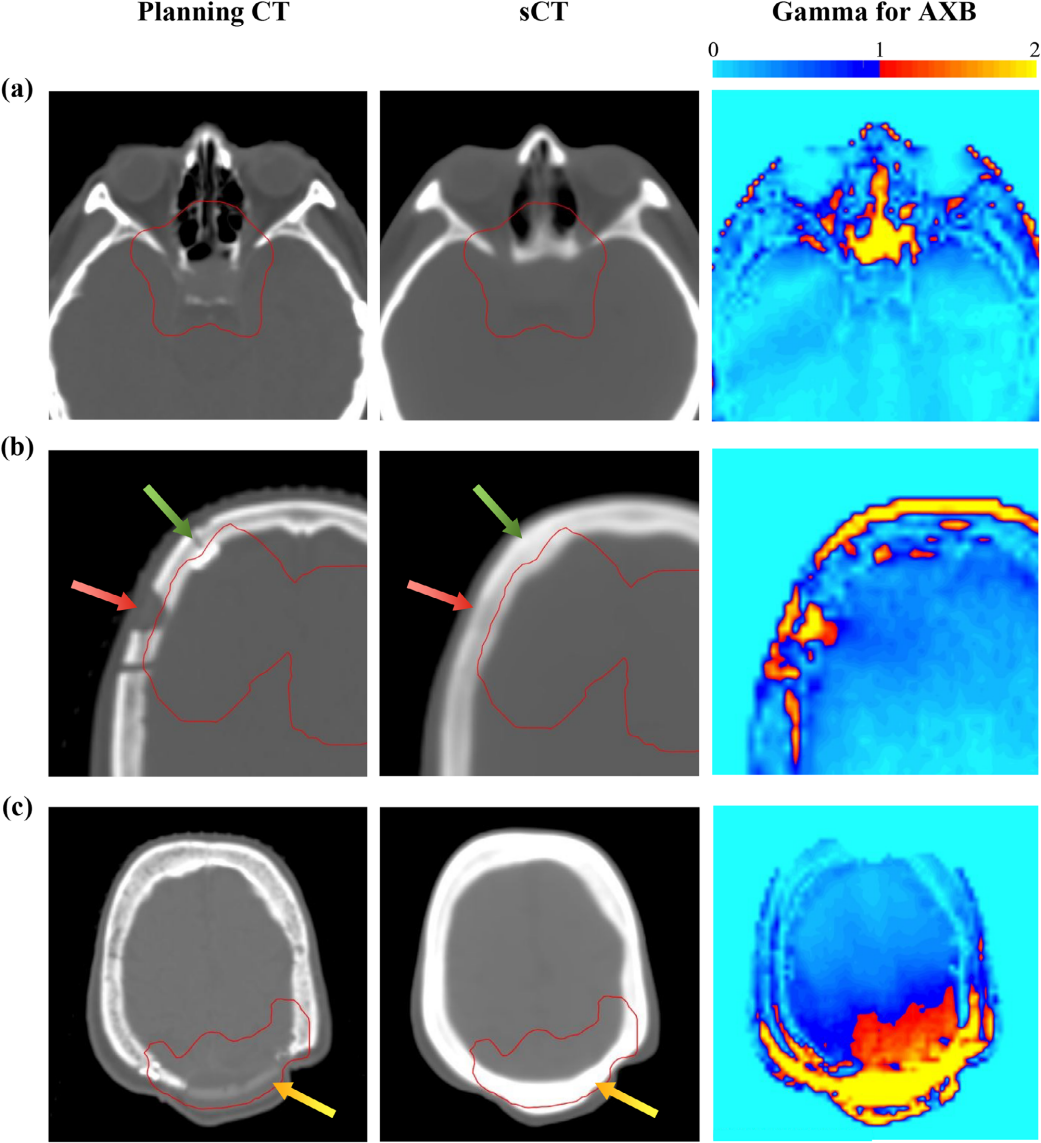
The planning CT (left), sCT (middle), and the 1%/1 mm global gamma distribution in entire imaged volume with a 10% low‐dose‐threshold for AXB (right) of three sample patients. The red contour in the planning CT and sCT outlines the PTV. (a) presents a case where the PTV is located near the sinus cavities. (b) presents a case with a post‐surgery drill hole and a metal screw (indicated by the red and green arrows, respectively), which were mislabeled as bone in the sCT. (c) presents a case with the use of synthetic material for skull contour restoration (indicated by the yellow arrow), which was mislabeled as bone in the sCT. PTV, planning target volume; sCT, synthetic CT.
